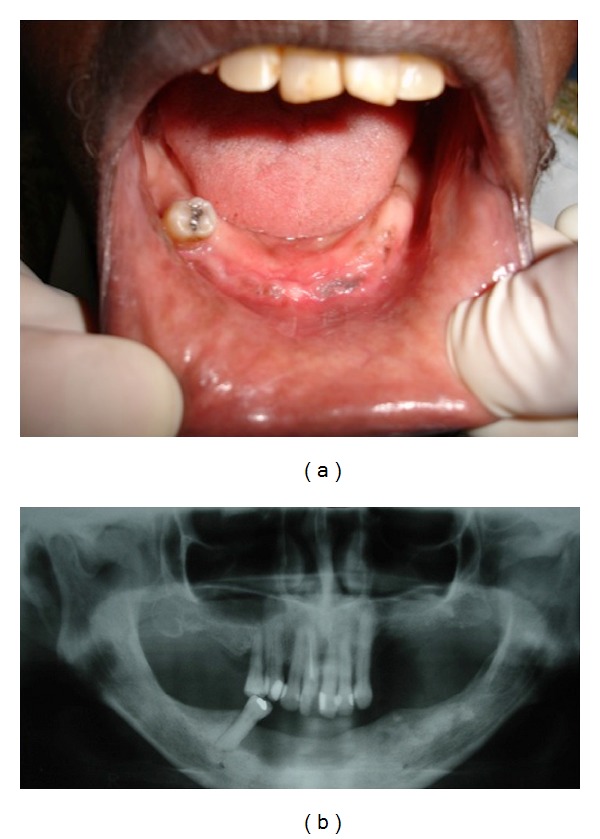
((a) and (b)) Clinical and radiographic aspects 30 days after surgery showing almost complete healing of the operated area.
